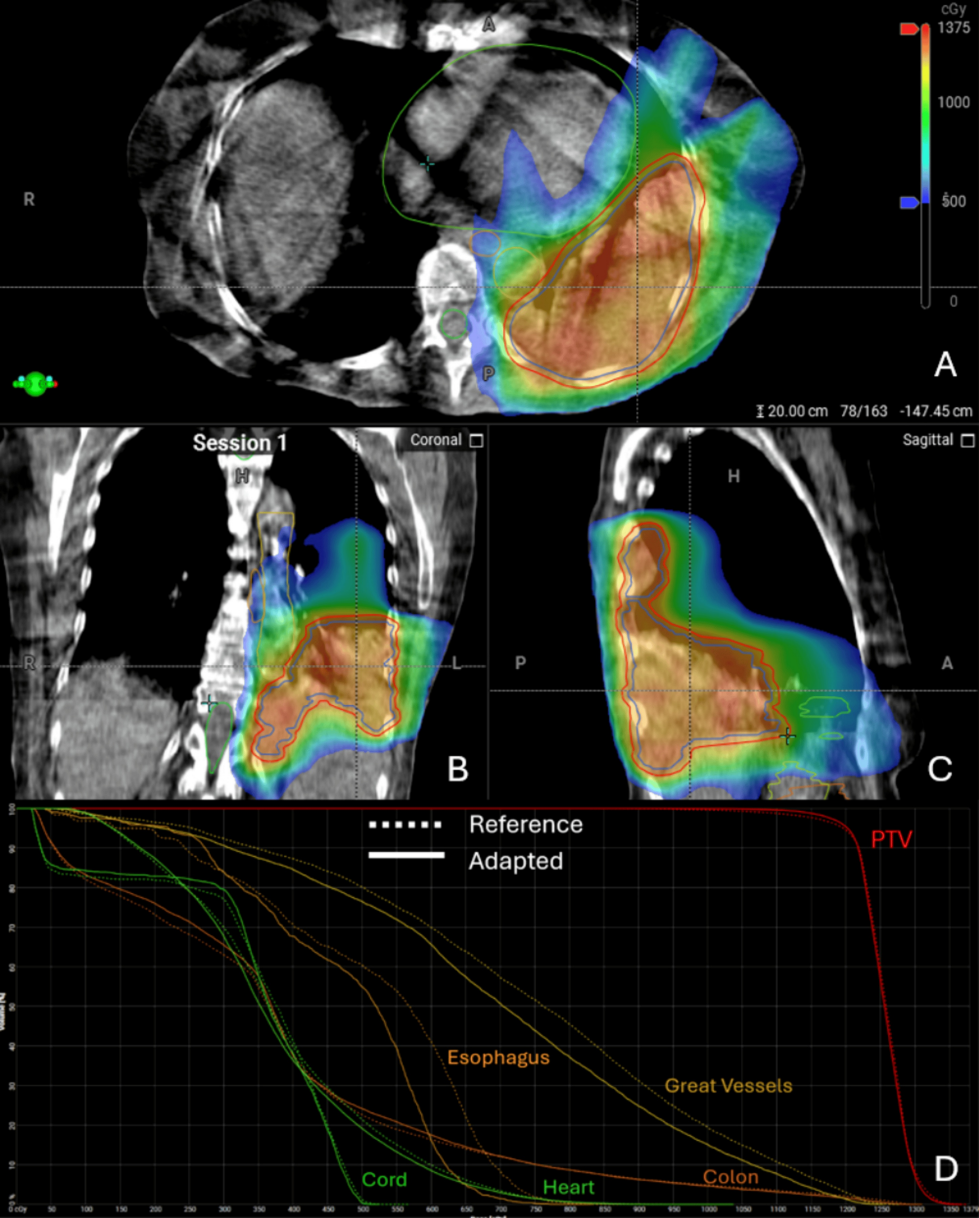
First Fraction of Online Adaptive Radiotherapy to a Bulky Thoracic and Abdominal Mass Cone-beam computed tomography (CBCT) and dose-volume histogram (DVH) from the first fraction of adaptive stereotactic body radiotherapy (SBRT). Dose colorwash with relevant target volumes and avoidance structures contoured on Ethos platform (Varian Medical Systems, Inc., Palo Alto, CA, USA) on axial (A), coronal (B), and sagittal (C) views. DVH (D) for both the selected adapted plan (solid line) and reference plan (dashed line) for planning target volume (PTV; red), great vessels (gold), colon (orange), esophagus (brown), heart (light green), and spinal cord (dark green).
